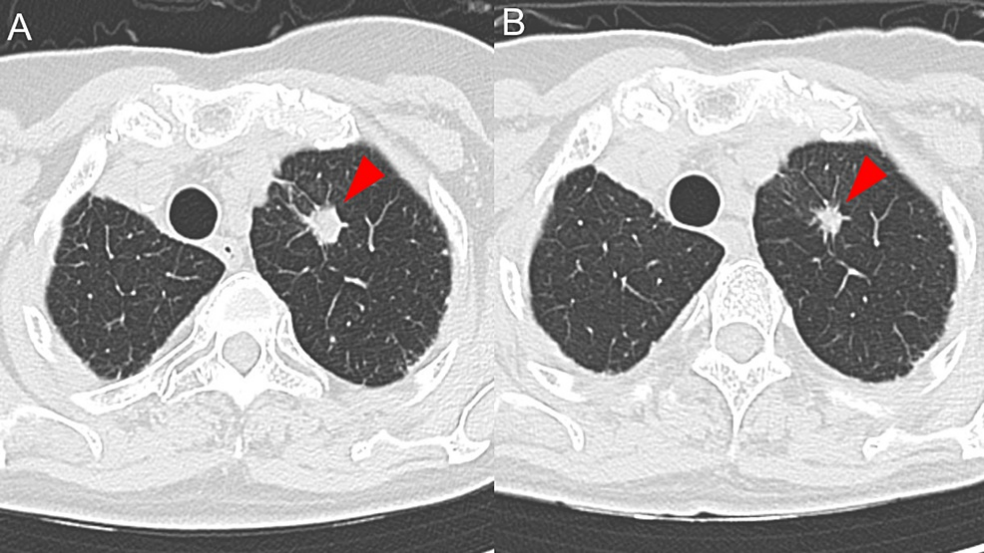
A series of chest computed tomography during trametinib treatment; at the initiation of the treatment (A) and after eight months of trametinib treatment (B), showing partial response.
